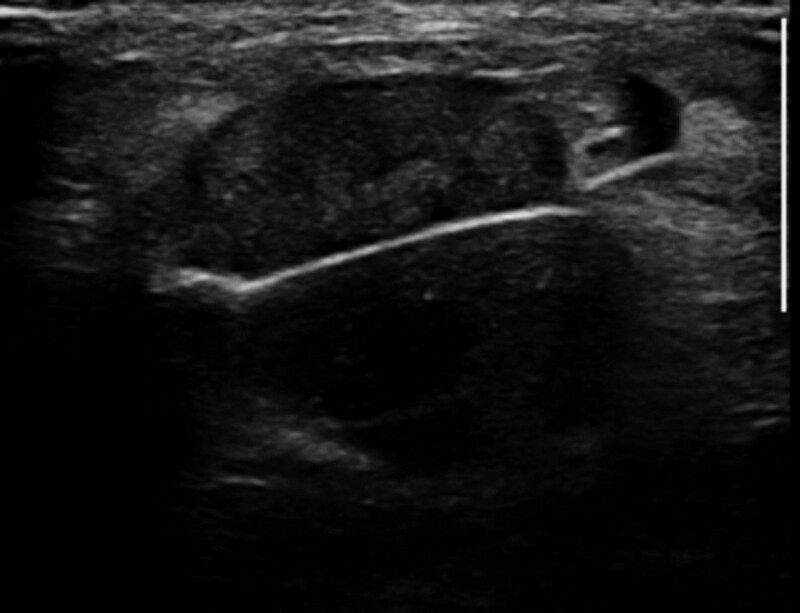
Ultrasound-guided core-needle biopsy revealed MEC of the breast, intermediate grade. MEC = mucoepidermoid carcinoma.
